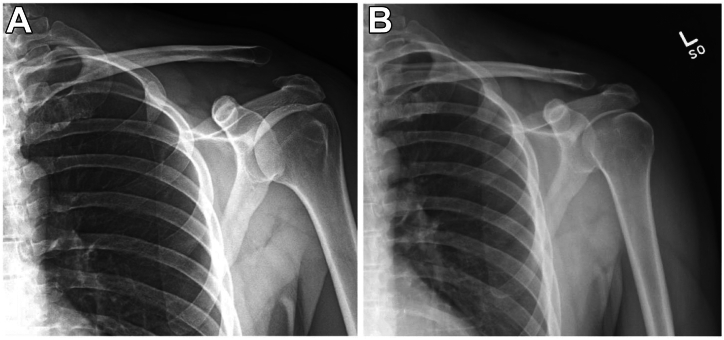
(A) Preoperative plain radiograph of the left shoulder demonstrating a type V acromioclavicular joint separation injury. (B) One-week postoperative plain radiograph of the left shoulder of the same patient demonstrating successful reduction of the acromioclavicular joint and restoration of the coracoclavicular interval.
